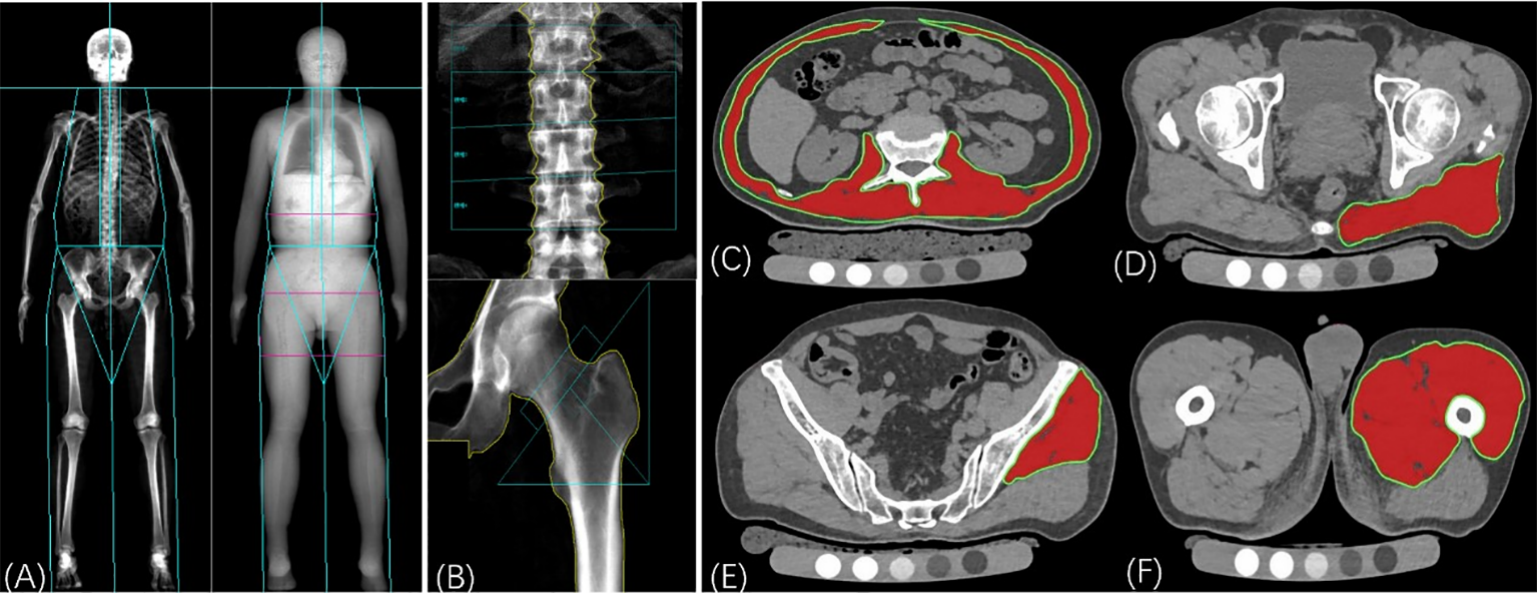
Dual-energy X-ray absorptiometry (DXA) and computed tomography (CT) assessments. (A): the whole body DXA scan for body composition analysis, mainly for the evaluation of muscle and fat mass. (B): Areal BMD was calculated by the DXA scan of the lumbar spine and left hip. (C)~(F): Muscle assessments with QCT including muscle density, muscle area, and intermuscular fat area. (C): Measurement of trunk muscle at mid-L2 level; (D): Measurement of cross-sectional area and mean computed tomography values of the gluteus maximus muscle at the level of the greater trochanter of the femur; (E): Measurement of the gluteus medius and minimus muscle at the third sacral level; (F): Measurement of the middle thigh muscle level. The muscle region is represented by the area highlighted in red. The area of intramuscular fat infiltration is obtained by subtracting the area of red ROI from the area of green ROI with the threshold segmentation method.
